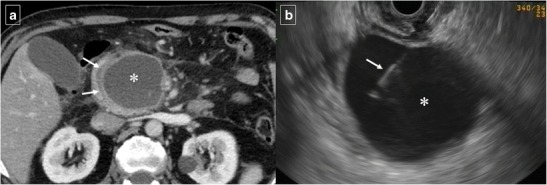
Duodenal compression by pancreatic pseudocyst in a 69-year-old man. a Axial contrast-enhanced CT reveals lateral displacement of the descending duodenum (arrows) by a pancreatic fluid collection (*). b Detail of an internal drain (arrow) guided by echoendoscopy to improve secondary food intolerance
